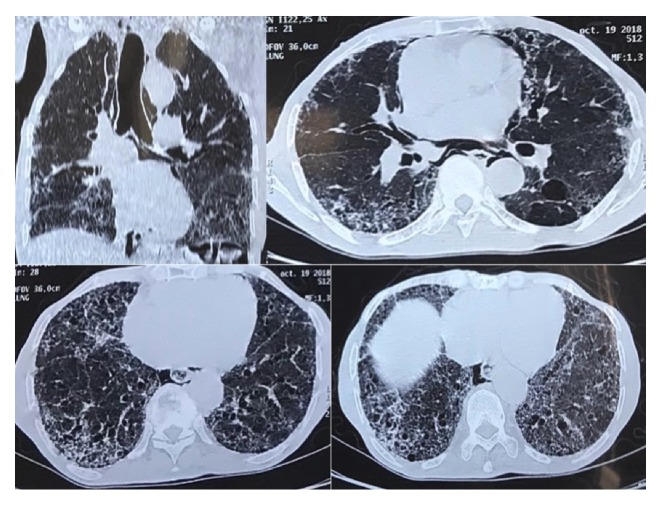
CT scan showing bronchiectasis, bullous emphysema, and fibrosis.
